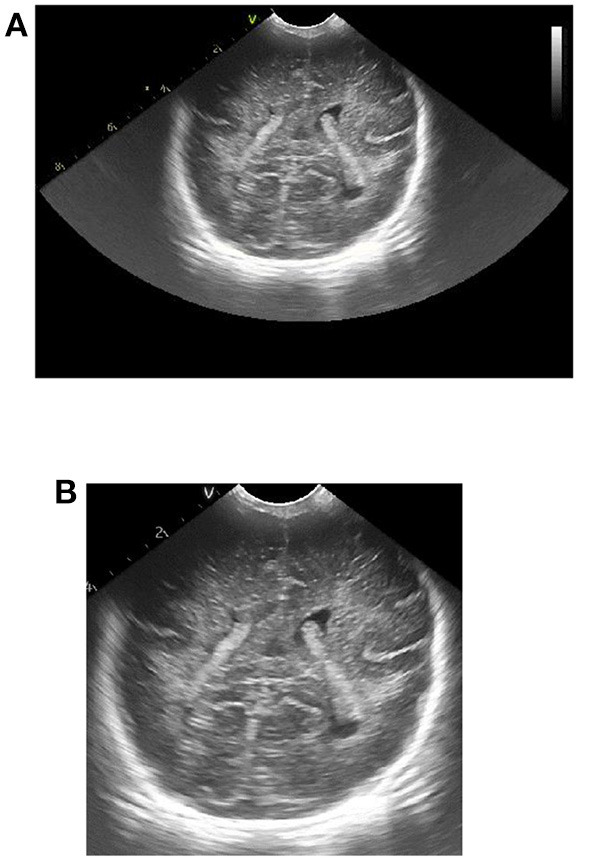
Effect picture of ultrasonic image clipping. (A) Ultrasound image before clipping, (B) cropped ultrasound image.
